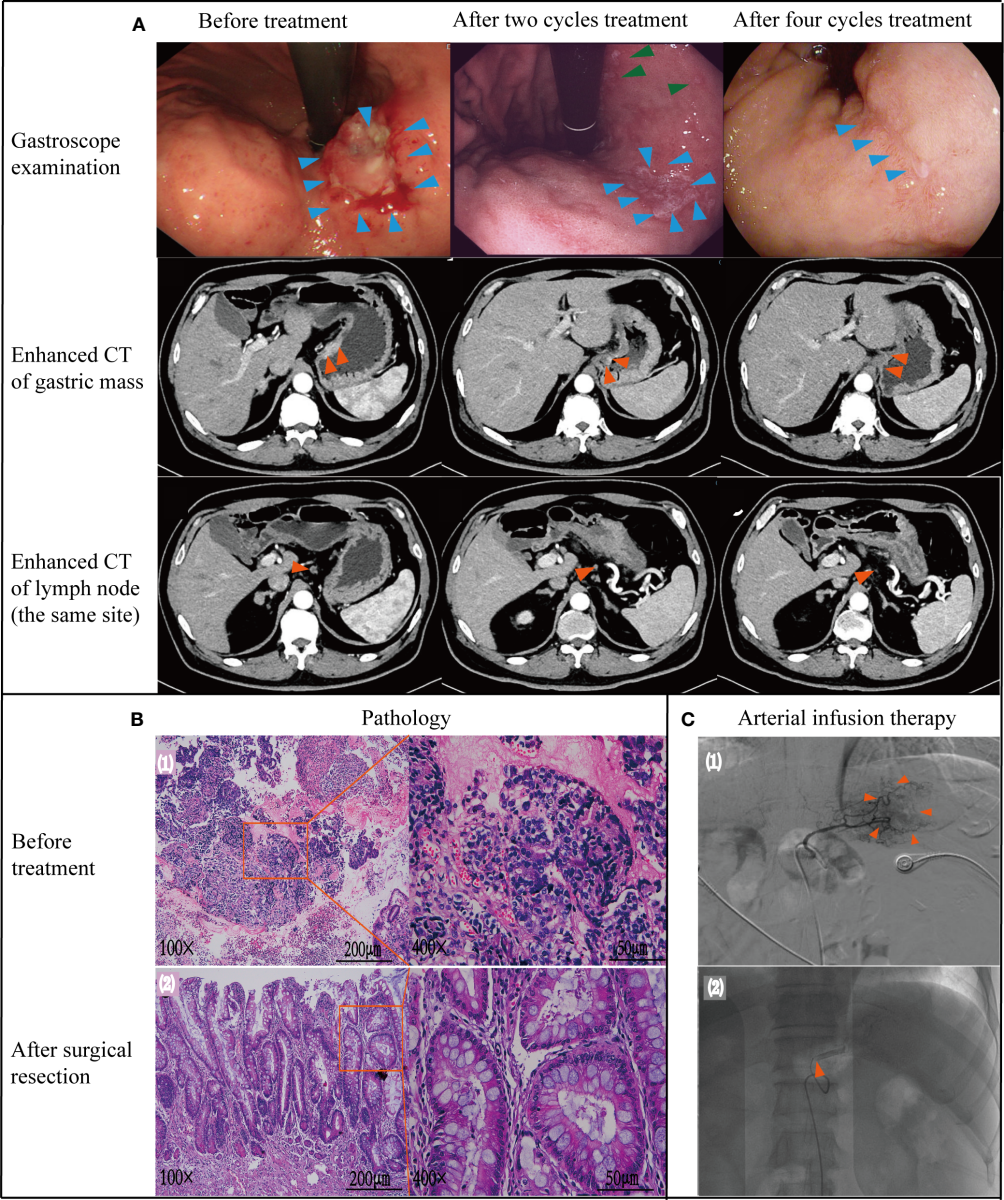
Case data of patient 2. (A) Gastroscope and imaging examination. Changes of tumor or lymph node in gastroscopy and enhanced CT before treatment, after 2 and 4 cycles treatment. (B) Pathology examination (1): biopsy pathology before treatment (adenocarcinoma); (2): pathology after surgical resection (no tumor cells found). (C) Treatment process under DSA (1): angiography showed that the feeding artery of the tumor was left gastric artery; (2): microcatheter tip placement location (in left gastric artery) Note: Figure 3A - green arrows. After 2 cycles of treatment, gastroscope also showed multiple small ulcers in the gastric body of patient 2, which was considered to be gastric mucosal reaction caused by high-concentration chemotherapy drugs in arterial infusion chemotherapy. After treatment with proton pump inhibitors, the patient's multiple ulcers improved and healed.
